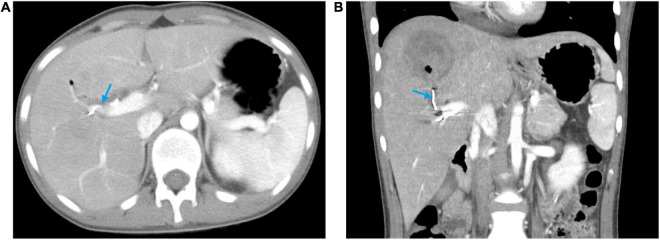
Abdominal contrast-enhanced computed tomography at discharge showing the pseudoaneurysm disappeared and the hyper-density coil (blue arrow). (A) Horizontal plane. (B) Coronal plane.
